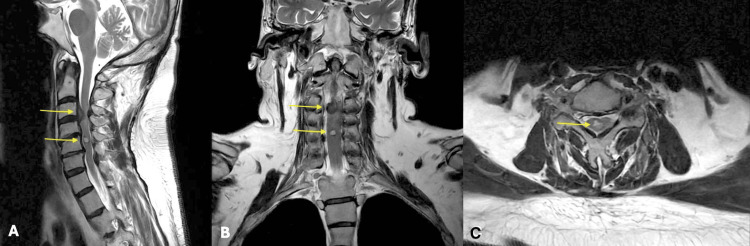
Preoperative T2-weighted MRI with sagittal, coronal, and axial reconstructions (A) Sagittal and (B) coronal T2-weighted MRI shows thickening of the posterior longitudinal ligament and disc fragment migrated into the canal of apparent C3-C4 origin. (C) Axial T2-weighted MRI shows an intramedullary lesion, central, at the level of C5, of oval morphology, heterogeneous signal, predominantly hyperintense with hypointense border, measuring 8×4×5 mm in its cephalocaudal dorsal-ventral and transverse axes, respectively (yellow arrows point to the lesions described). MRI: magnetic resonance imaging
